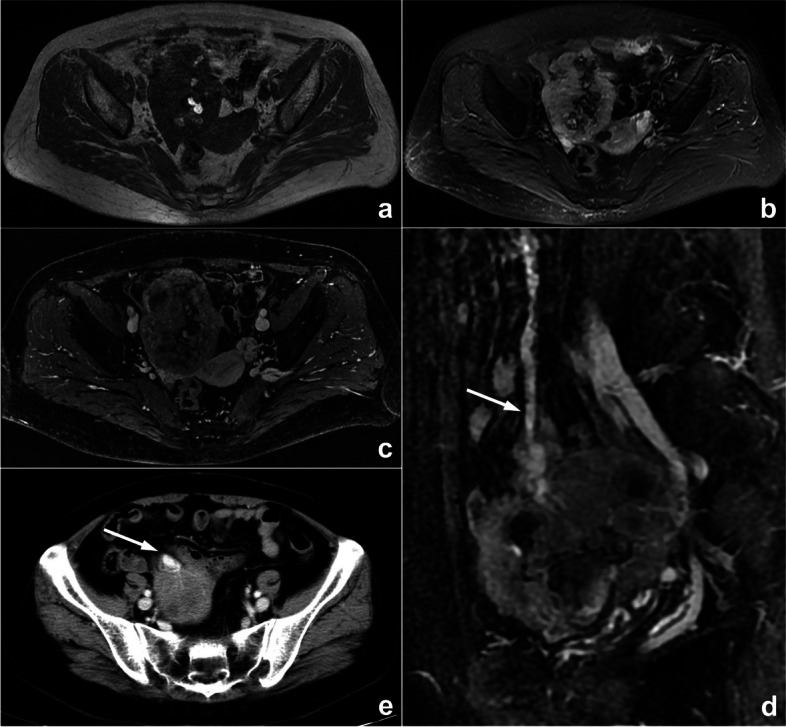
A palpable pelvic mass that was diagnosed as an ovarian tumor by the gynecologist in a 57-year-old woman who presented in gynecological clinics. MRI scan (a–c) showed a pelvic mass with hemorrhage-like confounding hyperintensity on T_1_WI and hypointensity on T_2_WI/FS internally. An enhanced scan represented heterogeneous enhancement. The mass was closely related to the small intestine, and blood supply by large mesenteric vessels (arrows) could also be observed on enhanced sagittal MRI (d) and axial CT (e) images, suggesting intestinal original disease. However, the radiologist mistook small intestinal stromal tumors as ovarian tumors because of the gynecologist’s diagnosis. This is classified as attribution bias
